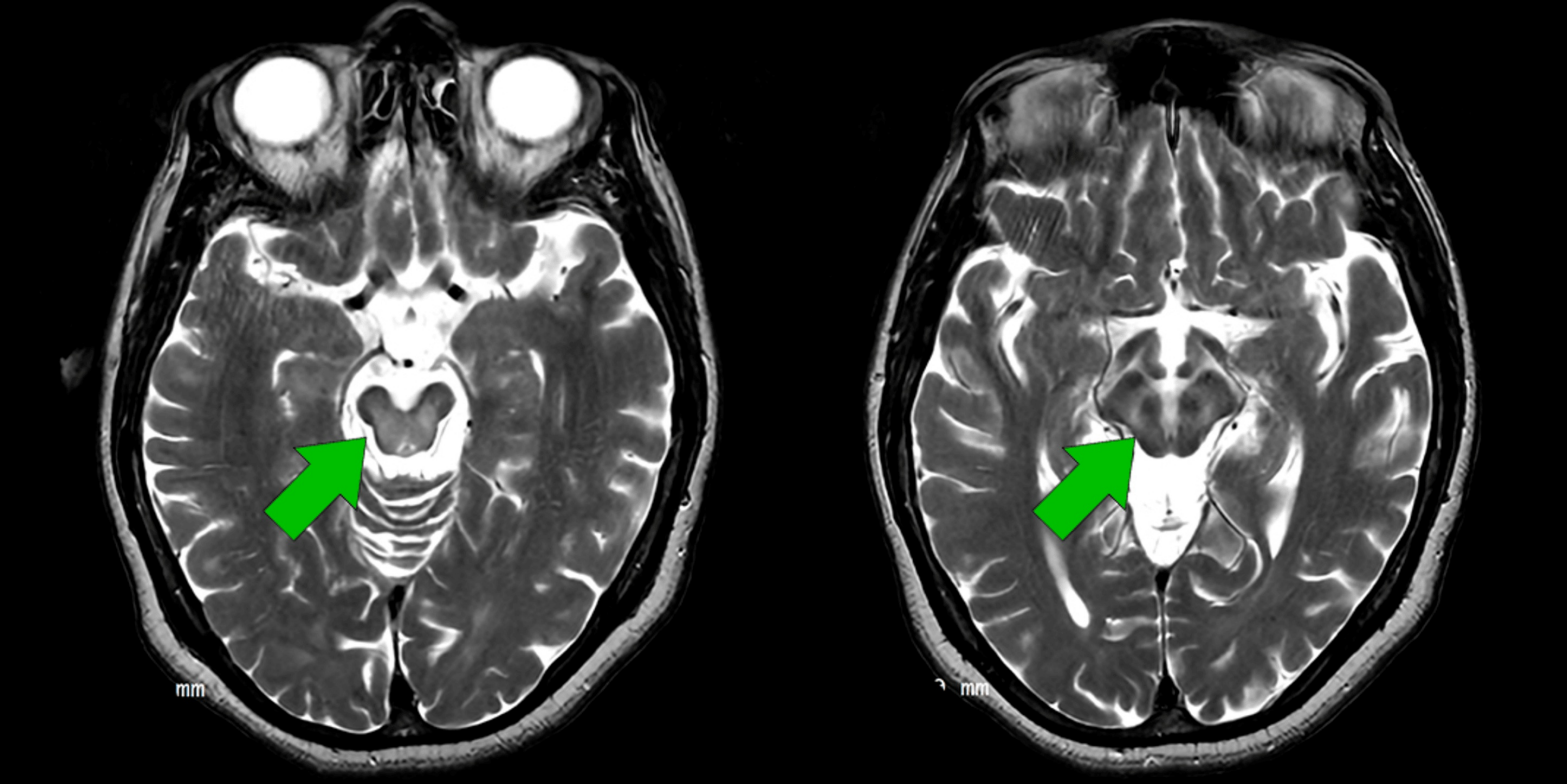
T2 sequence cuts of brain magnetic resonance imaging, with Giant Panda sign on the right
